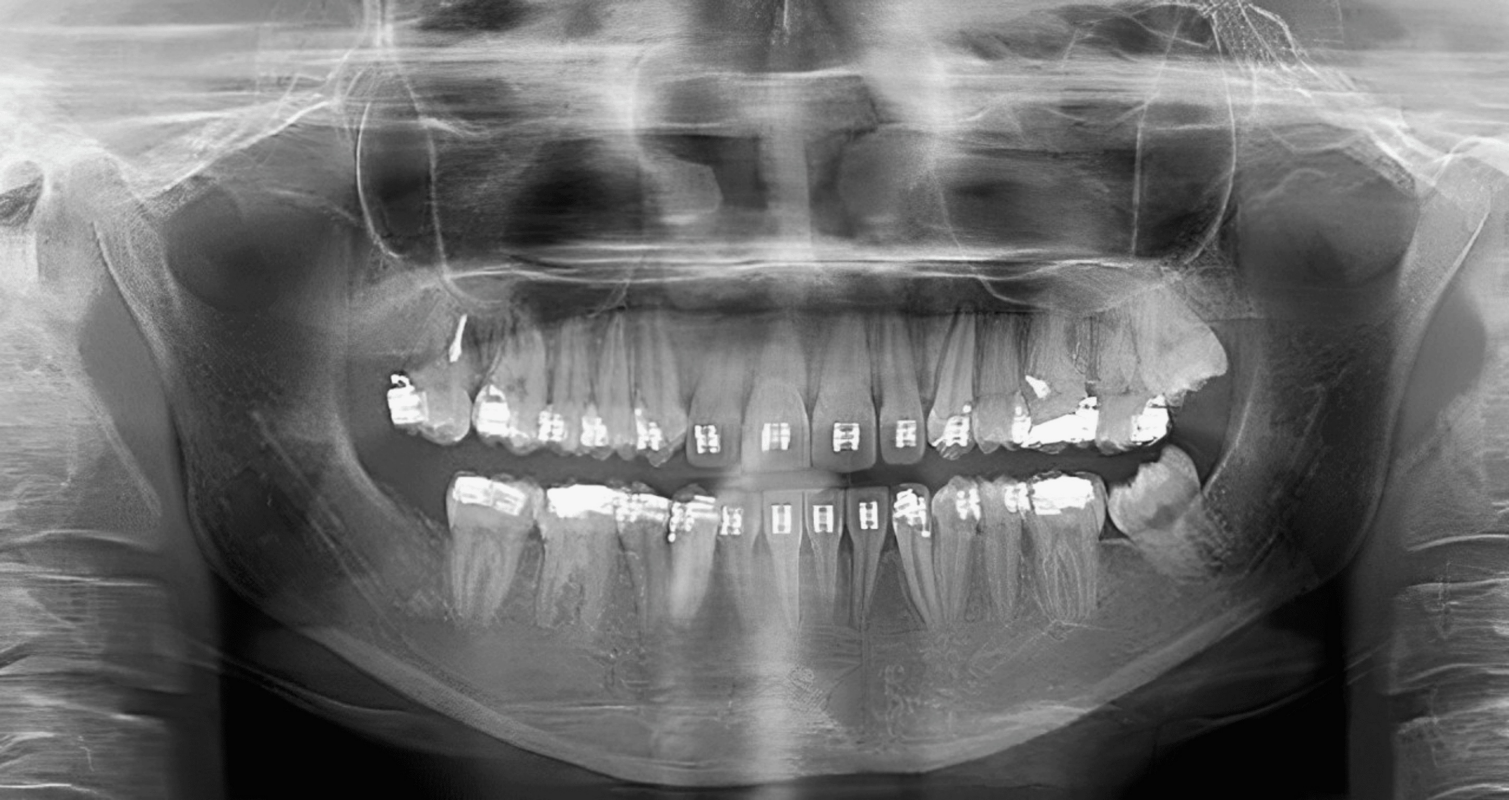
Postoperative OPG of the patient OPG: orthopantomography
